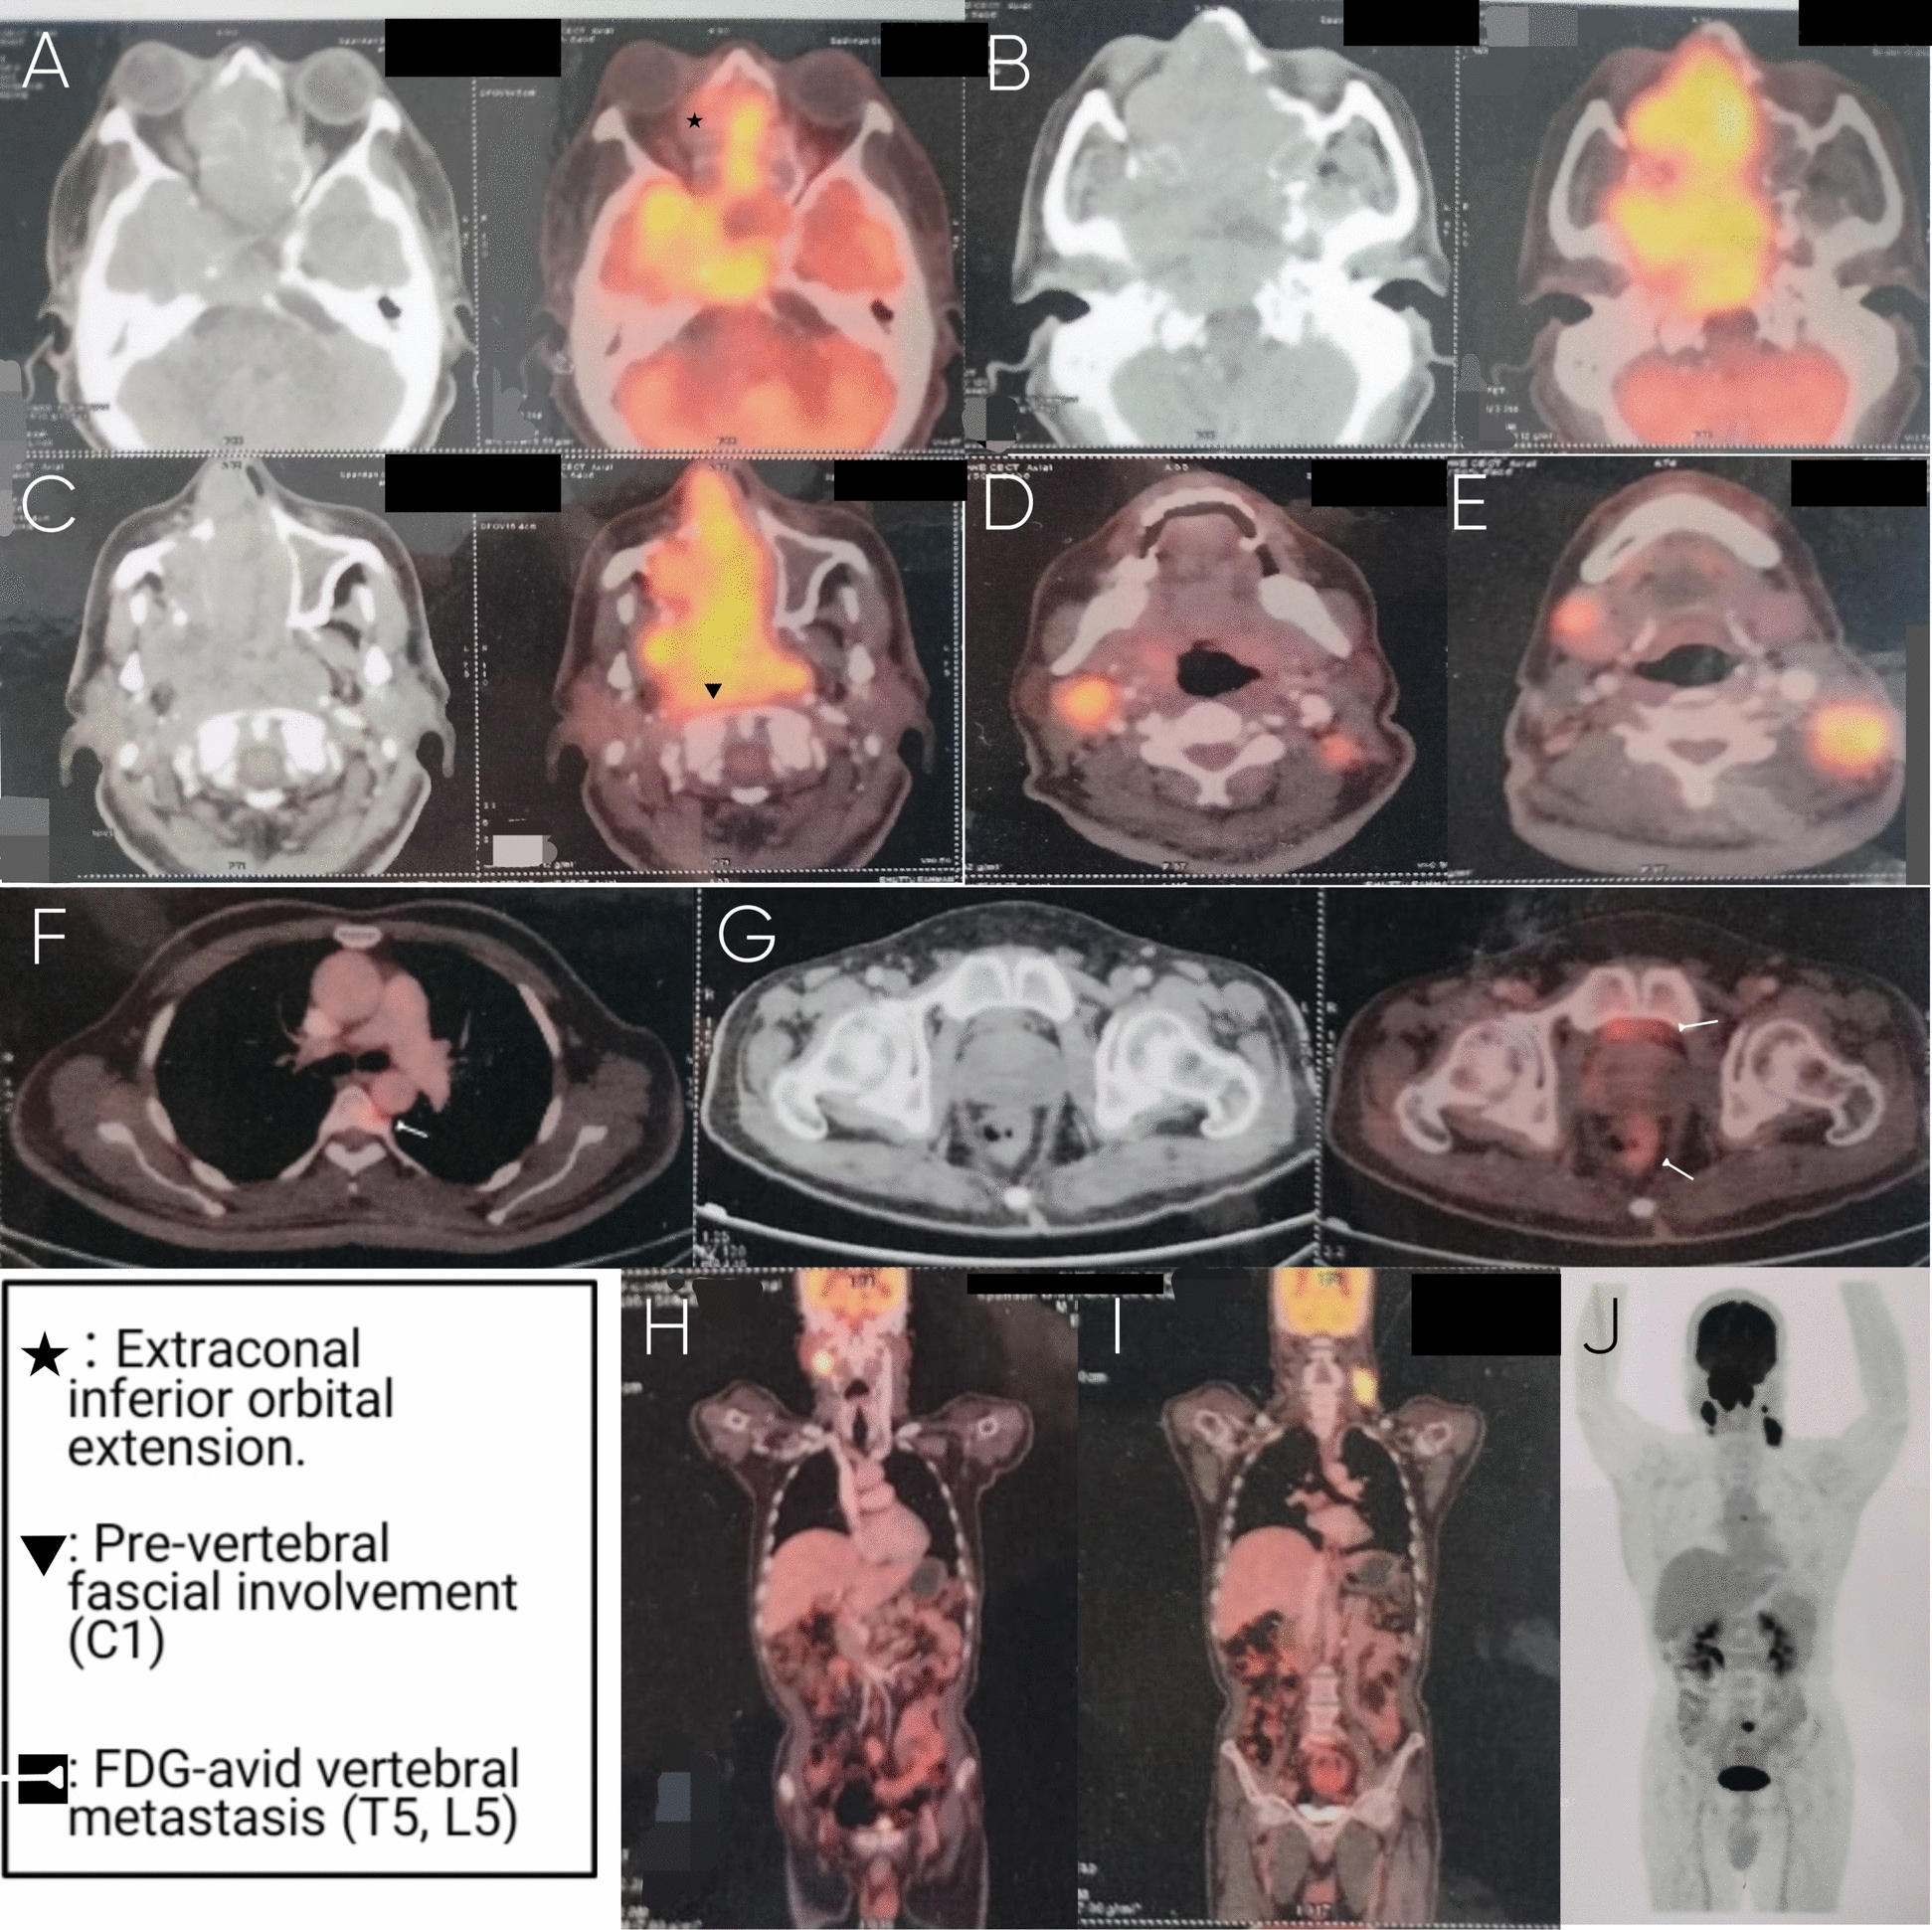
Whole-body ^18^F-FDG PET-CT demonstrating primary nasopharyngeal tumor and metastatic spread. Whole-body ^18^F-FDG PET-CT demonstrates an FDG-avid heterogeneously enhancing soft-tissue mass centered in the nasopharynx, involving both fossae of Rosenmüller, with anterior extension into the right nasal cavity, posterior extension into the prevertebral fascial space at the C1–C2 level, lateral extension into the right maxillary sinus and orbit, superior skull base erosion with intracranial extension, associated cervical lymphadenopathy, and distant skeletal metastases. A, B Axial CT and fused PET-CT images at the nasopharyngeal level show an intensely FDG-avid mass involving the nasopharynx bilaterally. C Axial CT image demonstrates posterior extension of the tumor with loss of the prevertebral fat plane at the C1–C2 level, along with nasal septal erosion and deviation toward the left. D, E Axial fused PET-CT images demonstrate FDG-avid metastatic cervical lymph nodes. F Axial fused PET-CT image of the thorax shows an FDG-avid lytic lesion involving the D5 vertebral body. G Axial CT and fused PET-CT images at the pelvic level demonstrate an FDG-avid lytic lesion involving the L5 vertebral body, and anterolisthesis of L5 over S1. H, I Coronal fused PET-CT images depict extensive metabolically active primary disease with nodal and skeletal metastases. J Maximum-intensity projection image demonstrates the overall distribution of FDG-avid disease
